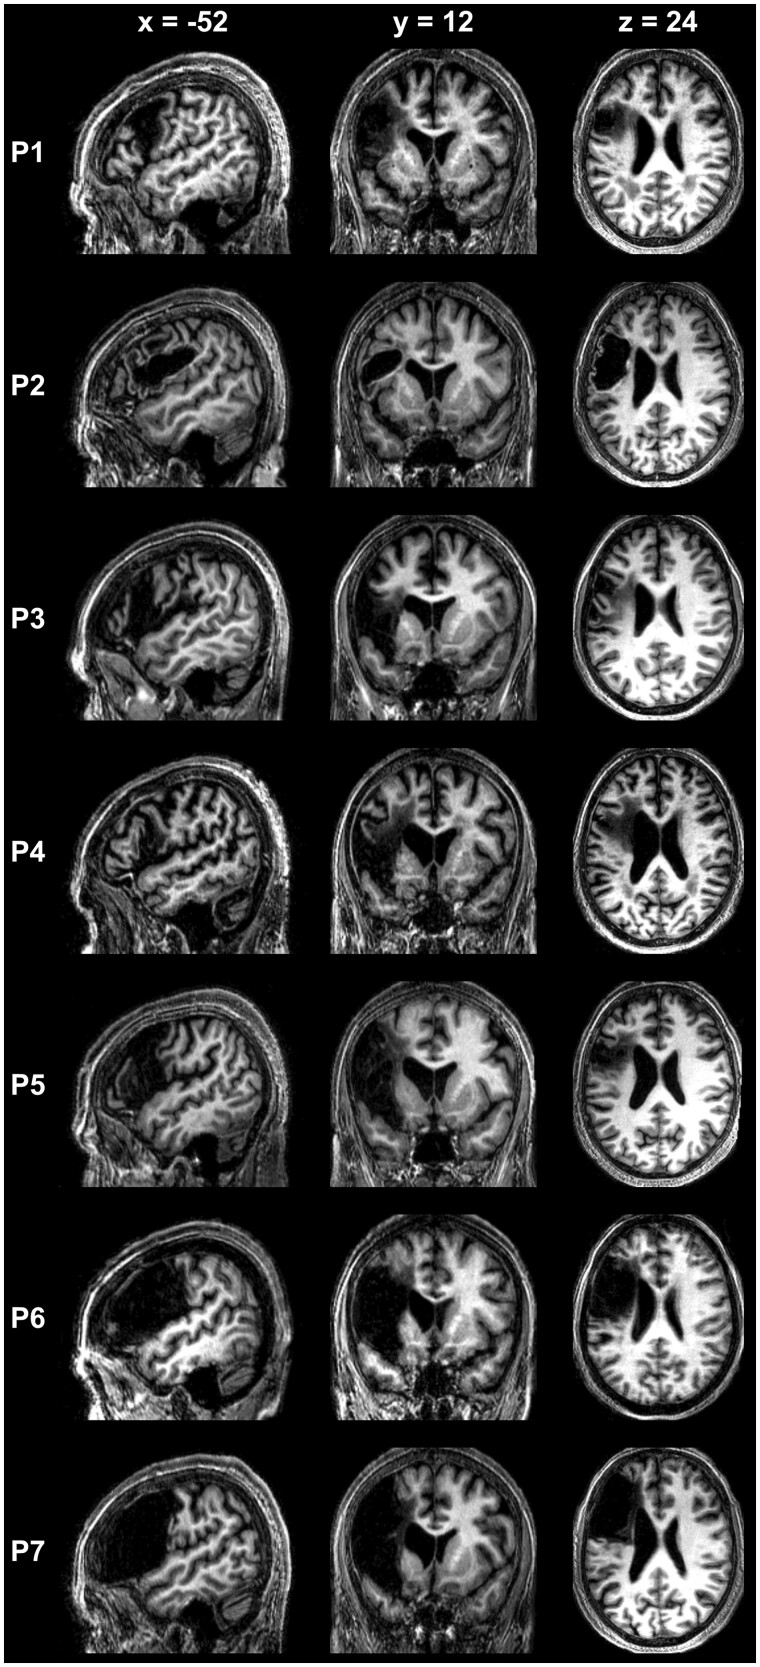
Spatially normalized T_1_-weighted MRI scans of seven patients with LpOp damage. Sagittal, coronal and axial views showing the location and extent of stroke damage in the seven patients of interest with LpOp damage. The scans are sorted from top to bottom by the size of the patient’s lesion (smallest lesion at the top and largest lesion at the bottom). In the PLORAS database, the following identifier was assigned to each patient: P1 = PS1414, P2 = PS0454, P3 = PS0005, P4 = PS0255, P5 = PS0426, P6 = PS0419 and P7 = PS0241.
